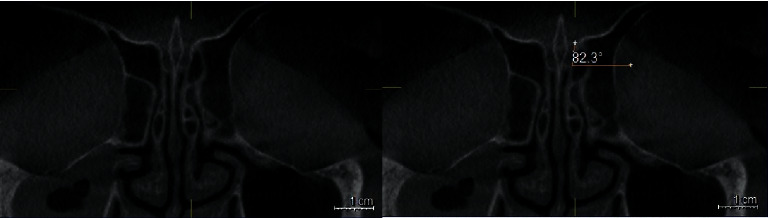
Coronal plan of CBCT demonstrating type I Gera classification on the left side.
